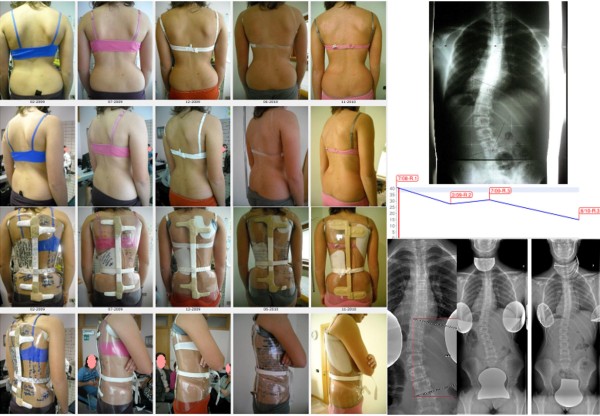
Second case report: adolescent thoraco-lumbar scoliosis patient still in treatment. On the left: posterior (first line), and sagittal (second line) aesthetics, and the brace in use (posterior - third line; lateral - fourth line) of all evaluations (apart the first visit) are reported from left (oldest) to right (last one). On the right, top-down, left-right direction: all x-rays of this case report of a patient still in treatment, and the graph with obtained results. C.S. has been evaluated the first time in July 2007, presenting with the first x-ray showing a thoraco-lumbar left scoliosis of 41° (Risser 1): fusion had been proposed but refused. She started treatment with the Sforzesco brace 23 hours per day and SEAS exercises 3 times a week (45' per session): after 6 months she was 28° (Risser 2). Treatment continued 6 months 22 hours per day, then with a 2 hours progressive weaning every 6 months. At the last x-rays after 2 years of treatment, performed after 8 hours without the brace, she was improved to 15° (Risser 3). Now she is wearing the brace 14 hours per day.
